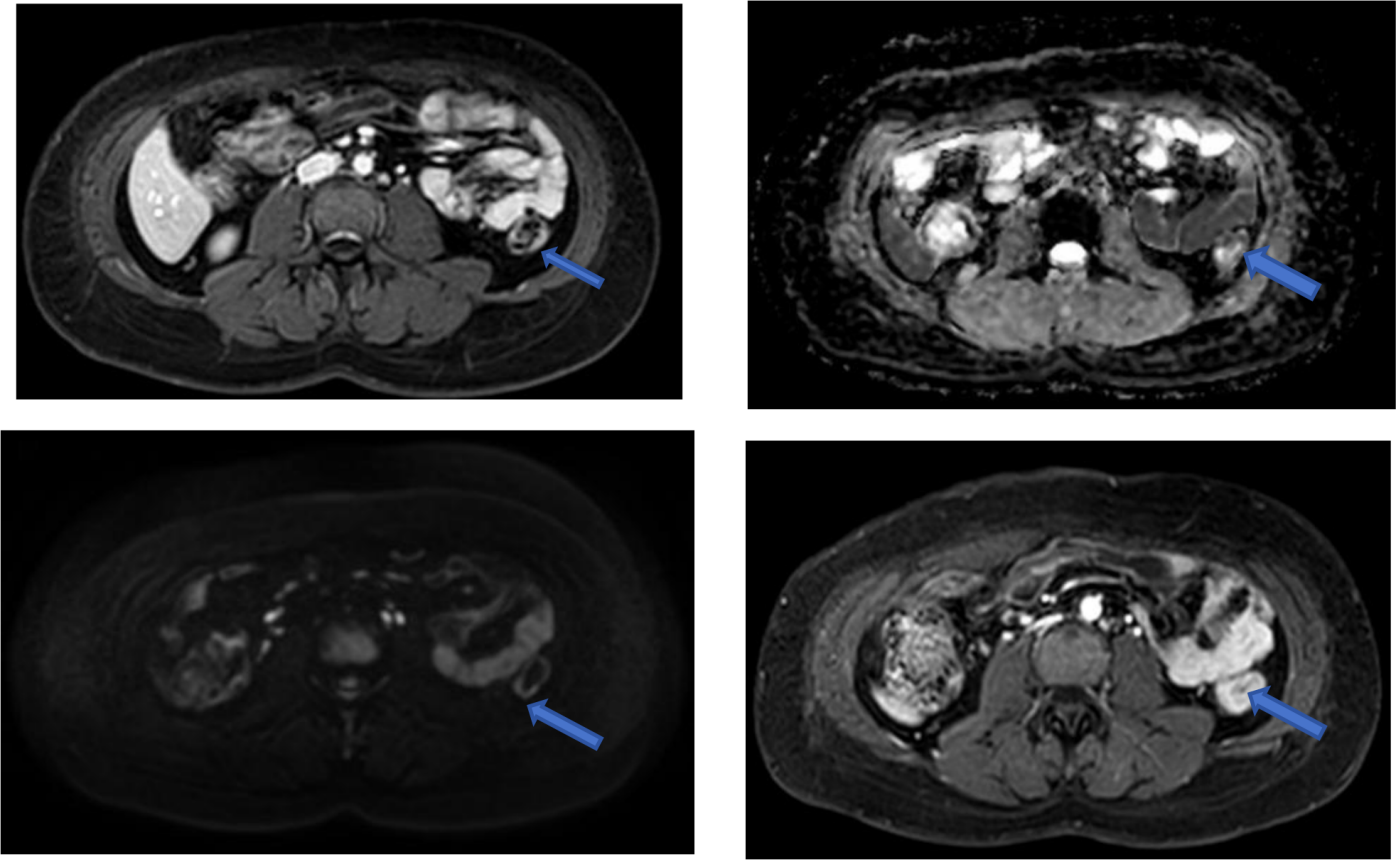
MRI exam on July 4, 2024:Blue arrows mark the tumor locations.
